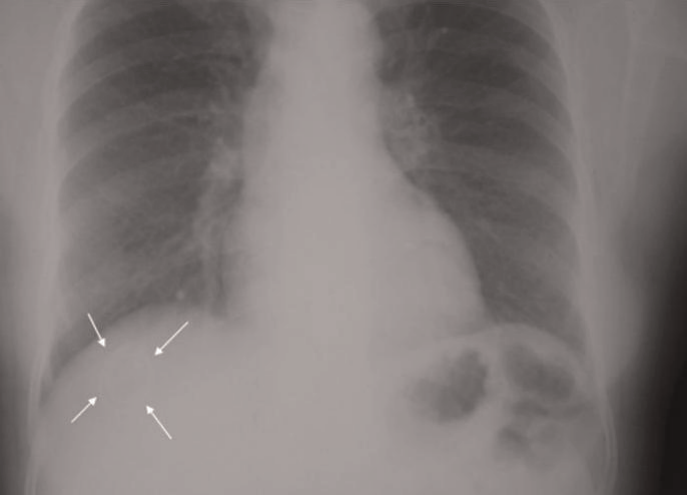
Chest X-ray LL showing the radioopaque IUD in the right subdiaphragmal region.
